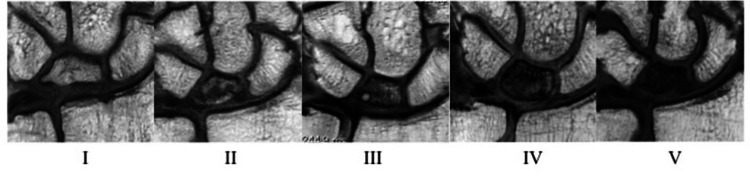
MRI grading system using the T1 or PDW coronal image On this scale, with respect to the signal intensity of the lunate, Grade I was considered almost normal; Grade II indicated localized regions of slightly decreased signal intensity; Grade III indicated a slight, generalized decrease in signal intensity; Grade IV represented low signal intensity with regions of high or isointense signals, and Grade V indicated generalized low signal intensity MRI: magnetic resonance imaging; PDW: proton density-weighted
